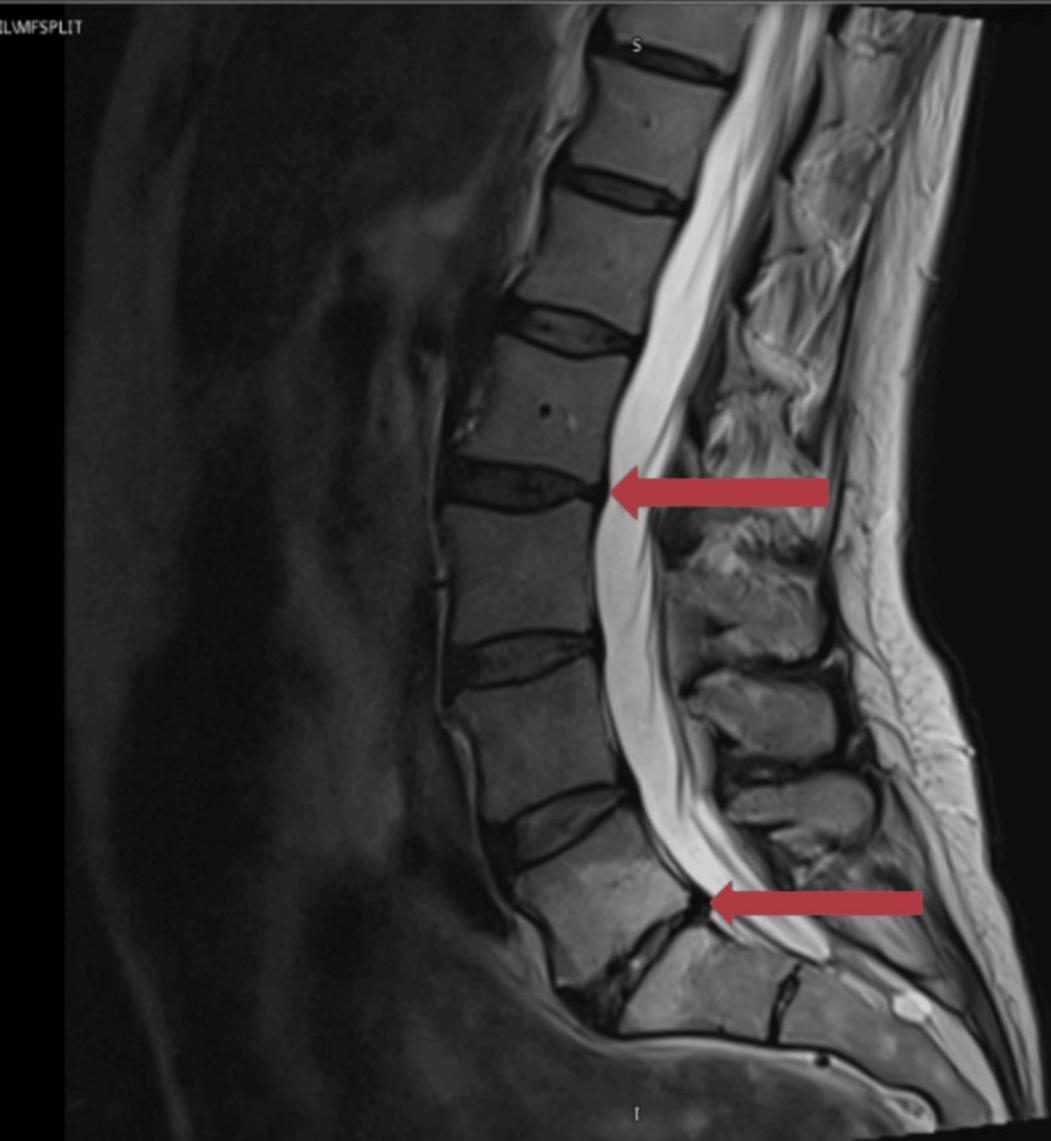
MRI image of the spine (lumbar and sacral region). The red arrows indicate areas where there are minor degenerative changes with some loss of disc height, but there is no substantial vertebral misalignment. There was no frank neural impingement and no other pertinent positive findings identified. The report concluded that there was no discrete structural cause for the reported clinical presentation identified.
